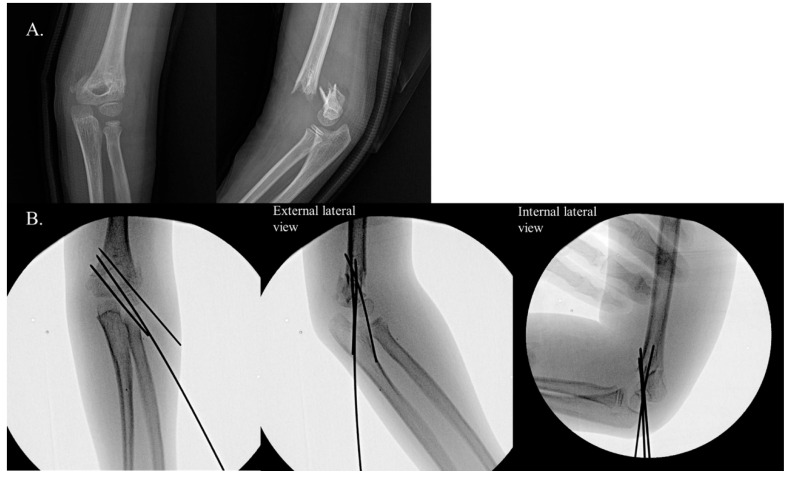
Example of negative internal rotational stress test (IRST) results in a 9-year-old boy with a Gartland type III supracondylar fracture. Panel (A) shows the preoperative radiographs. Panel (B) depicts C-arm images demonstrating a negative IRST. Good bone contact and well-maintained reduction are observed in both the anteroposterior (AP) and external lateral views. Notably, the reduction was maintained even in the internal stress lateral view.
